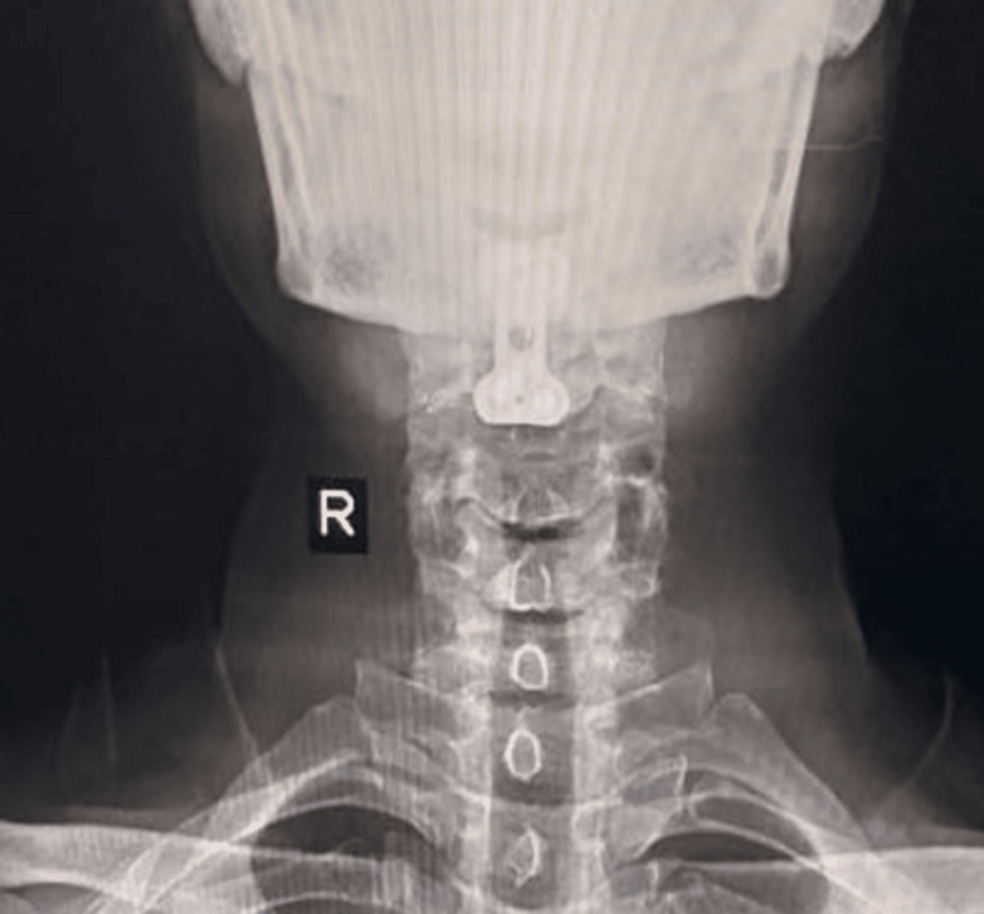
Post-operative X-ray anteroposterior view: one-month follow-up.
